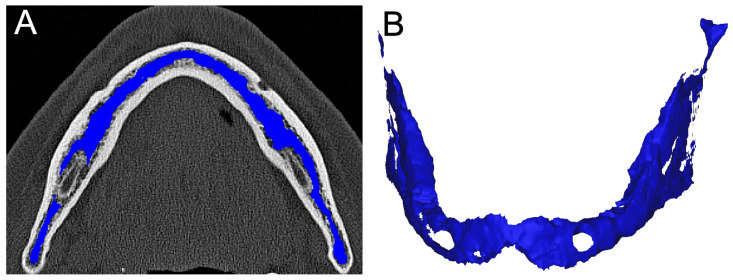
Segmentation example for mandibular BMD calculation. (A) An axial reformation of the CT dataset and (B) a 3D reconstruction with bone marrow colored blue. This figure visualizes which anatomic regions form the mandibular BMD.
